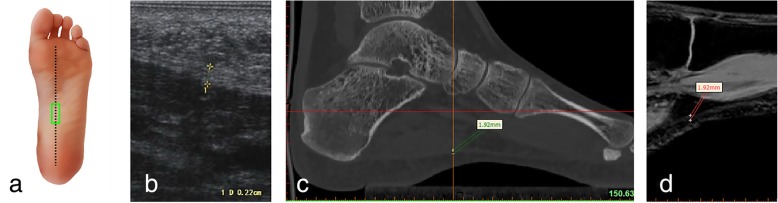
Ultrasound, CT and MRI measurements of the arch of the plantar fascia (longitudinal section) a Ultrasonic probe is placed (green panel). b Ultrasonic measurement. c Measuring the thickness of the plantar fascia reconstruction CT images. d Measuring the thickness of the plantar fascia reconstruction MRI images
